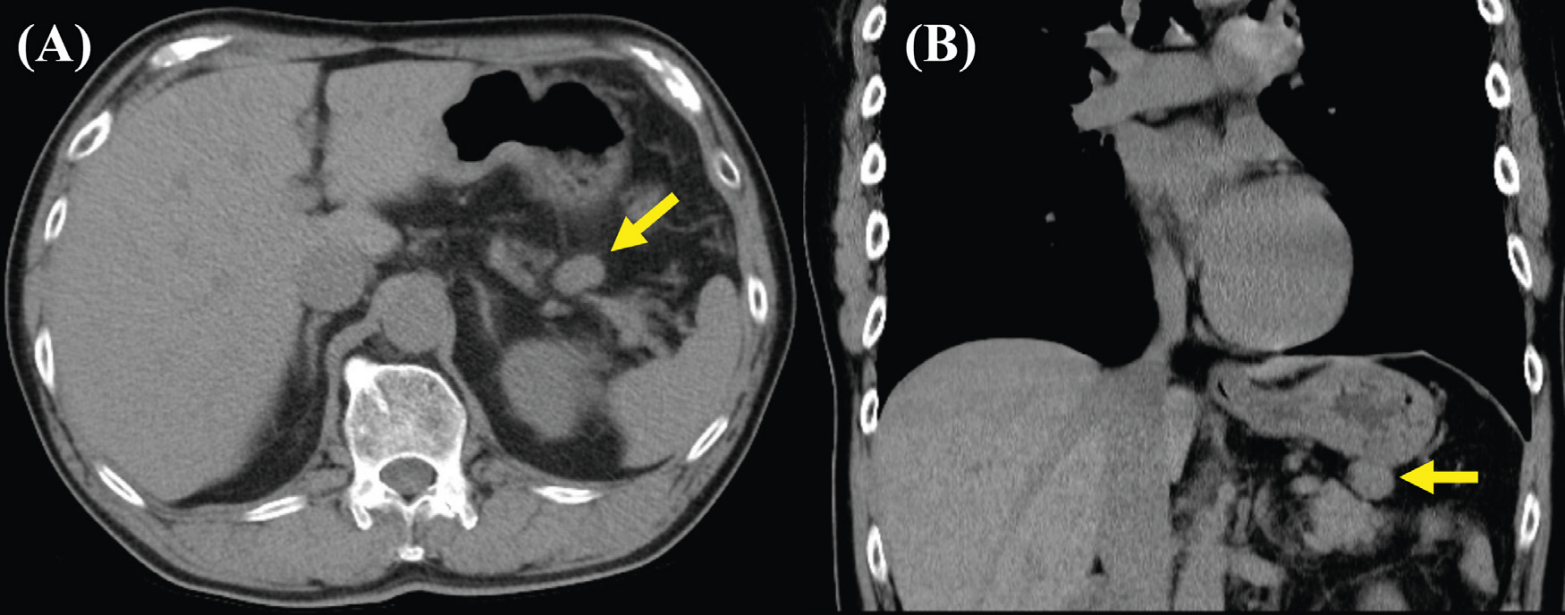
Abdominal CT findings Abdominal axial (A) and coronal (B) CT revealed well-defined round mass in the gastropancreatic region measuring 15 mm in diameter (yellow arow).
